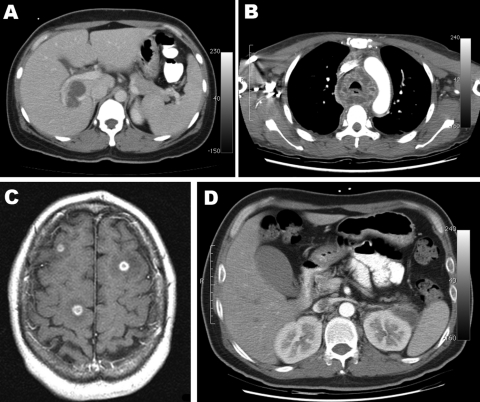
A) Computed tomography (CT) scan of the abdomen showing a liver abscess adjacent to the portal vein. B) CT scan of the chest at the level of the aortic arch showing mediastinum abscesses surrounding the trachea. C) Brain magnetic resonance imaging (T1 weighted, spin echo, with contrast) showing multiple intracerebral abscesses (smooth ring-enhancing lesions with surrounding vasogenic edema). D) CT scan of the abdomen of patient from panel C, showing a left perinephric abscess and thrombus.
